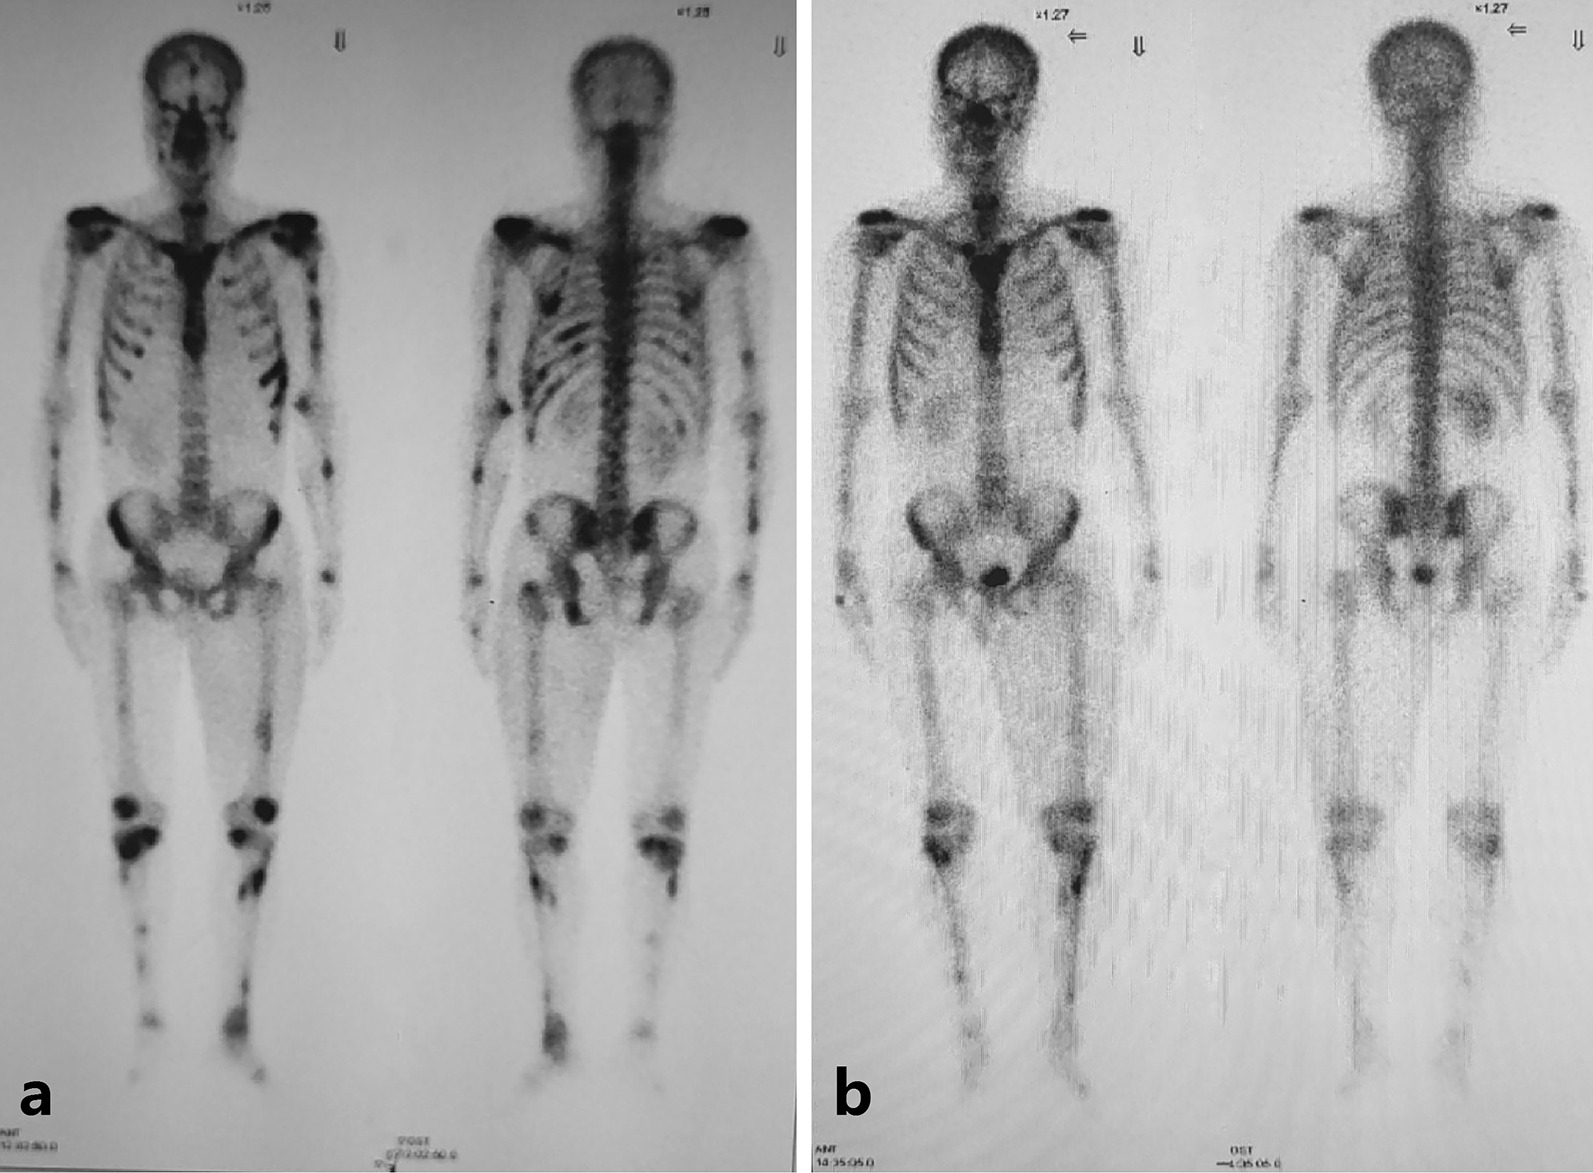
Findings after chemotherapy. Emission computed tomography (ECT) showed increased radioactive concentration by the clavicle, sternum, multiple ribs, vertebrae, right shoulder blade lower horn, pelvis, and long bones of the limbs. After chemotherapy (a), ECT showed reduced radioactive content in the primary bones (b)
